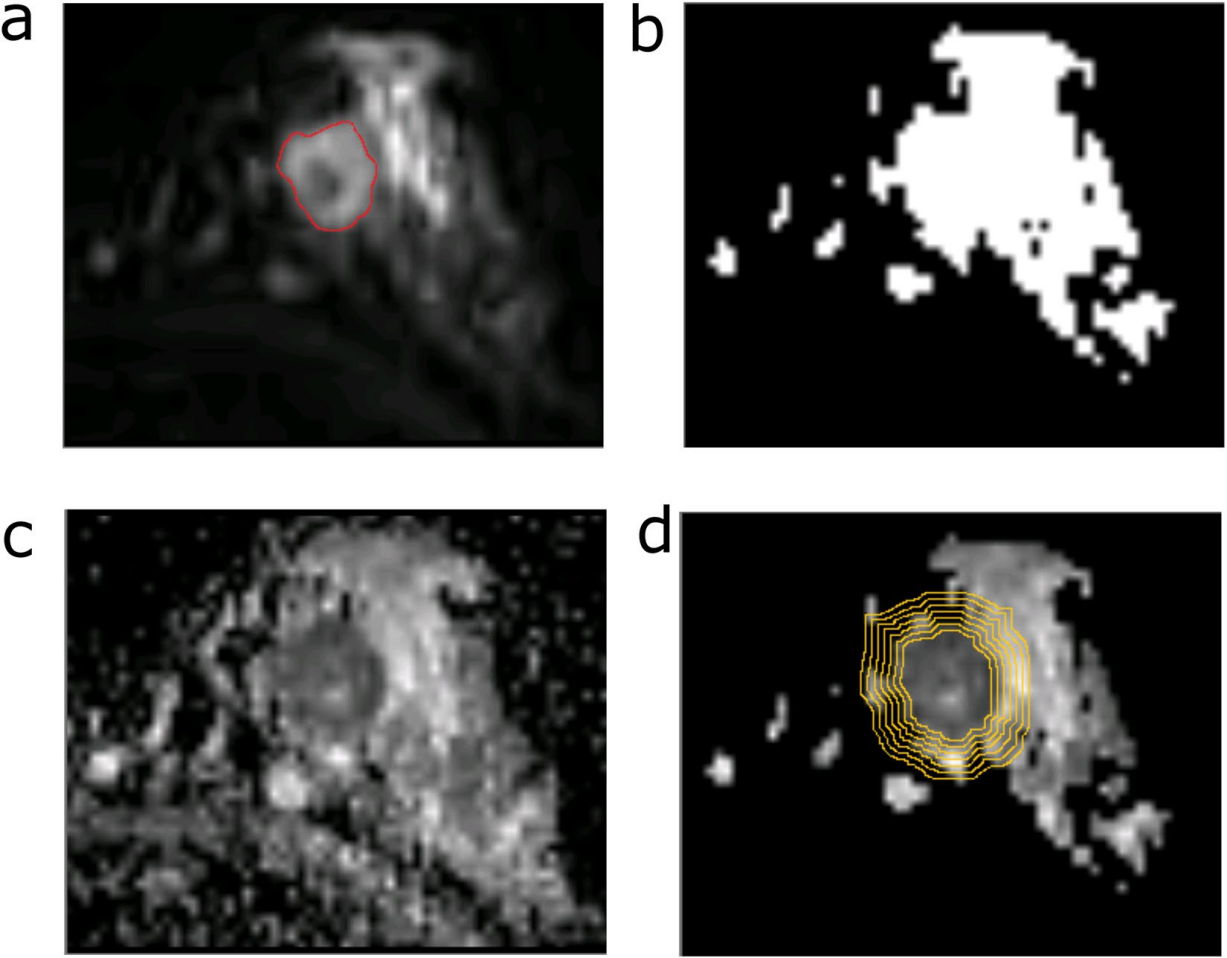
An example of a peritumoural stromal map in the left breast of a patient. (a) Diffusion-weighted image at b = 50 s/mm^2^ shows a mass with high signal intensity (red circle). (b) Fibroglandular tissue, excluding the fat from the breast, was segmented on the DWI b = 50 image. (c) ADC image of the breast. (d) The fibroglandular proximity map was applied to an ADC image to calculate the proximal peritumoural stromal ADC map.
